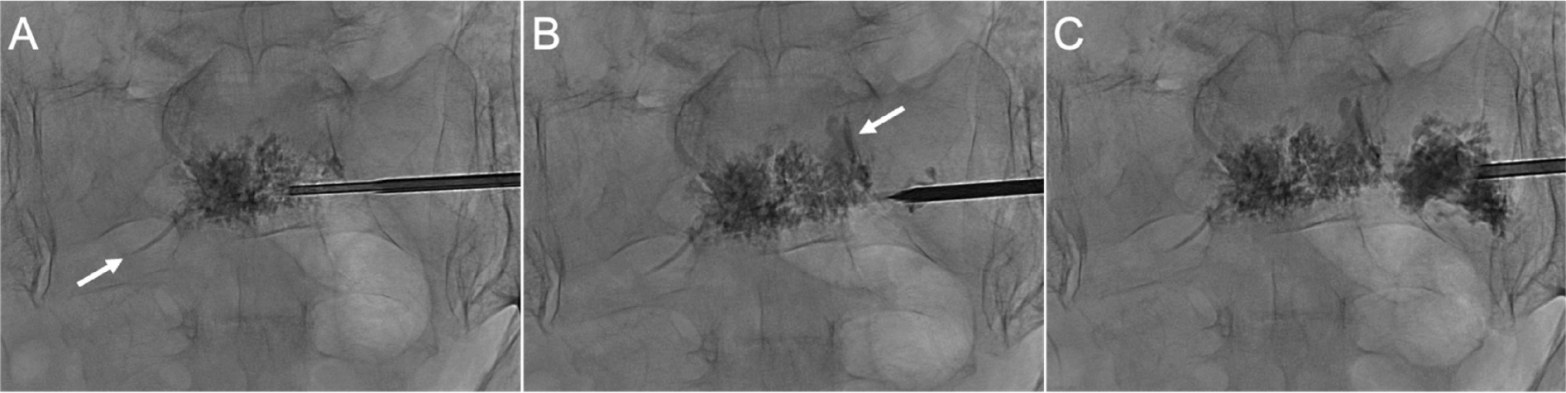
First application of the co-axial sequential injection technique involving a patient with insufficiency fractures involving the sacral body and right foramen. (A) PA radiograph after initial injection demonstrates cement in the sacral body and small presacral venous extravasation (*arrow*). (B) Subsequent injection around the foramen showed a small foraminal leakage (*arrow*). (C) Subsequent injection, following a period for cement curing, showed that the foraminal leak remained unchanged (*arrow*) without further propagation along the needle path. The foraminal leak was asymptomatic.
